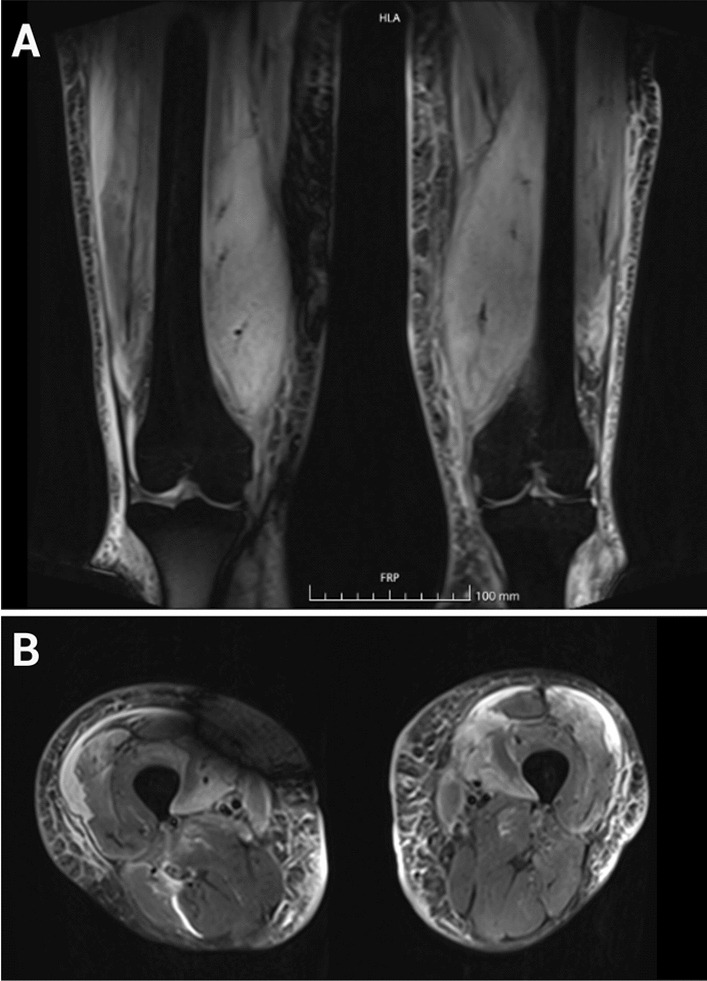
A, B Extensive bilateral symmetric muscle edema in the quadriceps muscle at initial presentation in an magnetic resonance imaging scan
